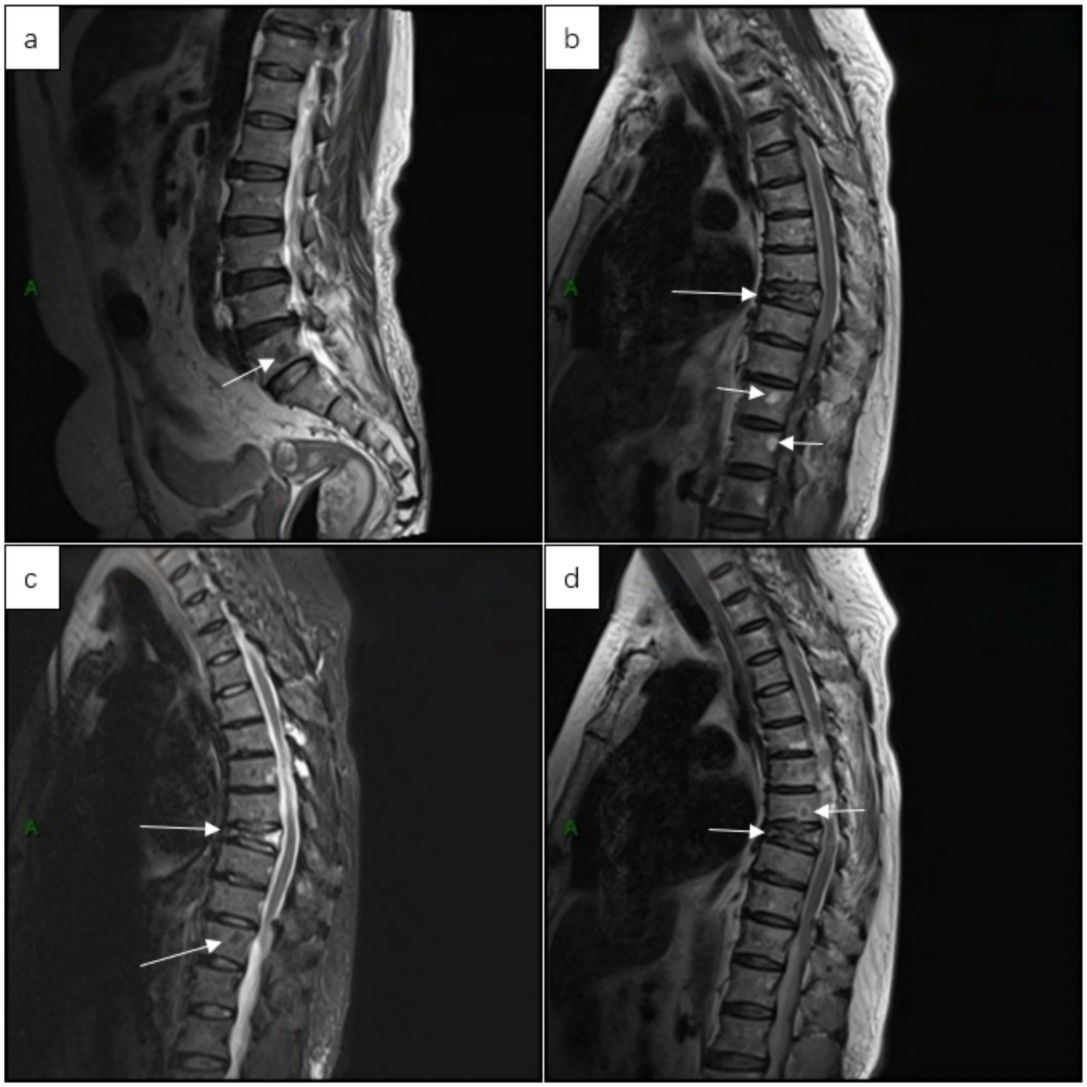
MRI of the spine demonstrating multifocal osseous lesions involving the lumbar spine (a) and thoracic spine (b–d), consistent with myelomatous infiltration, with partial compression deformities in several thoracic vertebral bodies.
